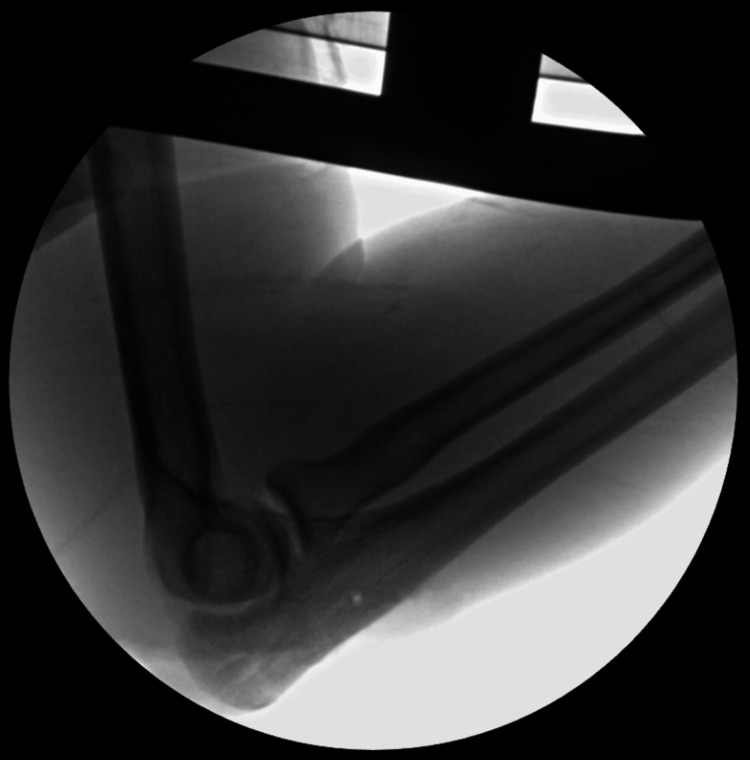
Intraoperative lateral X-ray image (fluoroscopy) of the right elbow.
